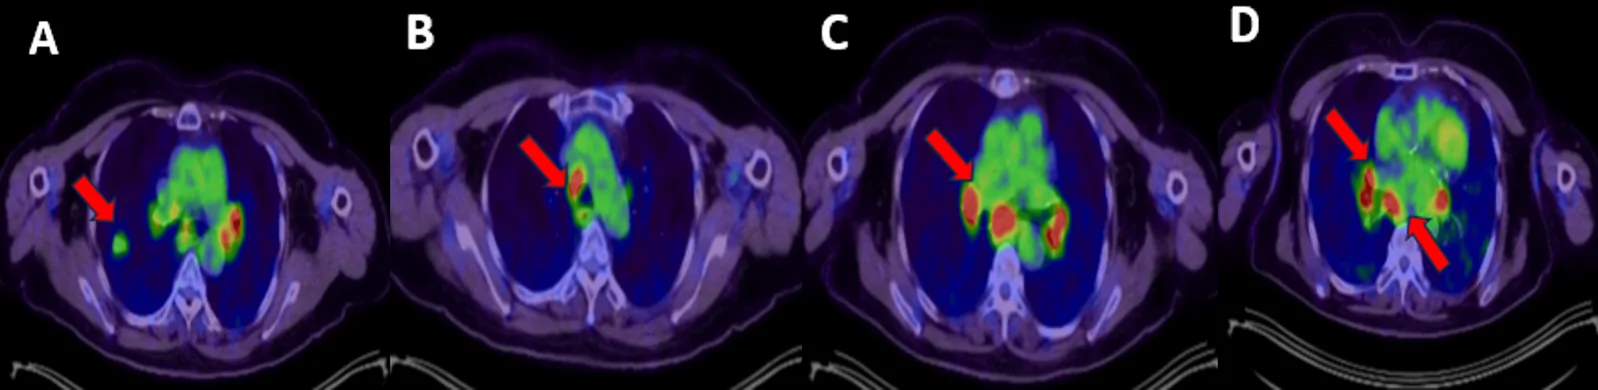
Faint uptake (SUVmax: 2.3) within a part-calcified 18 mm right upper lobe nodule (A) and increased metabolic uptake in mediastinal and hilar lymph nodes (B-D) SUVmax: maximum standardized uptake value
